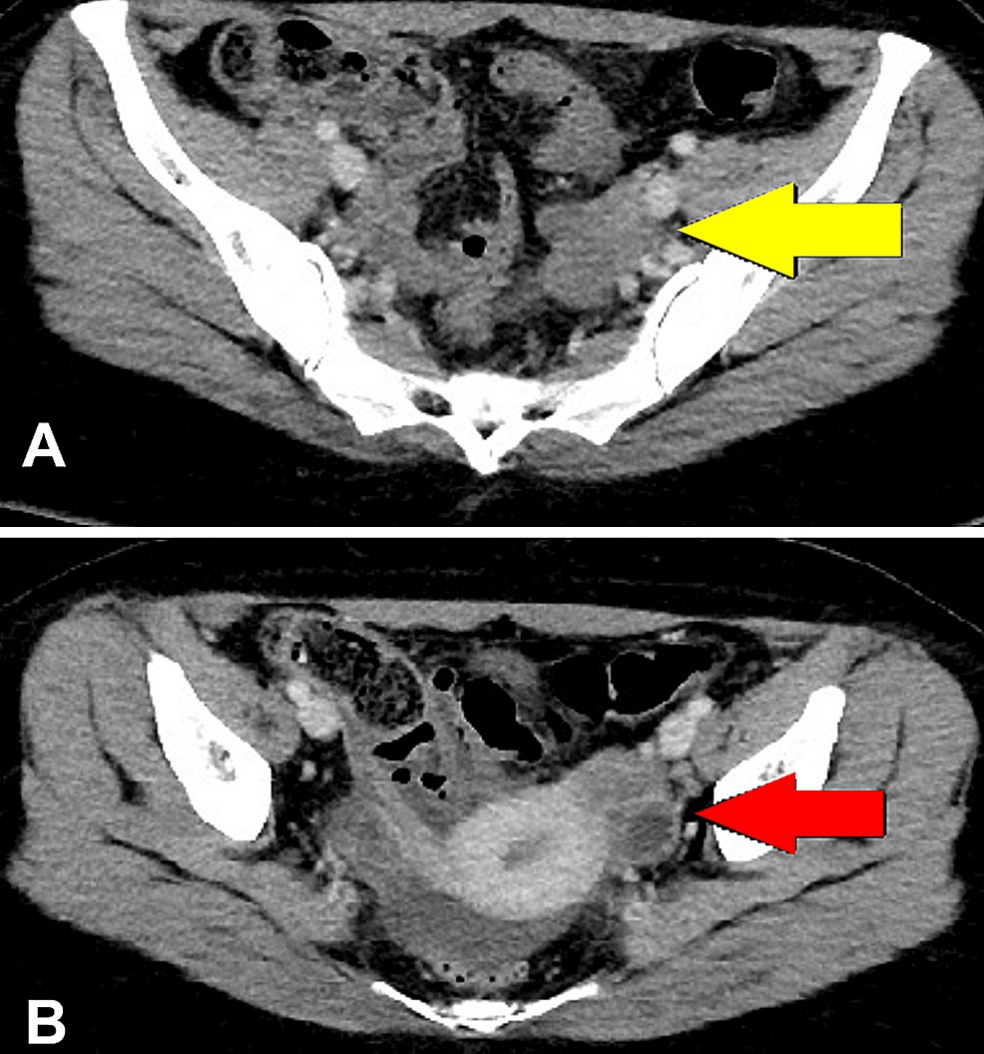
(A) Post-surgery contrast-enhanced CT scan axial image showed bulky left ovary (yellow arrow). (B) Axial contrast-enhanced CT scan through pelvis post-surgery of the same patient showed a small cyst in the left adnexa with enhancing wall (red arrow). A small amount of free fluid is also seen.
